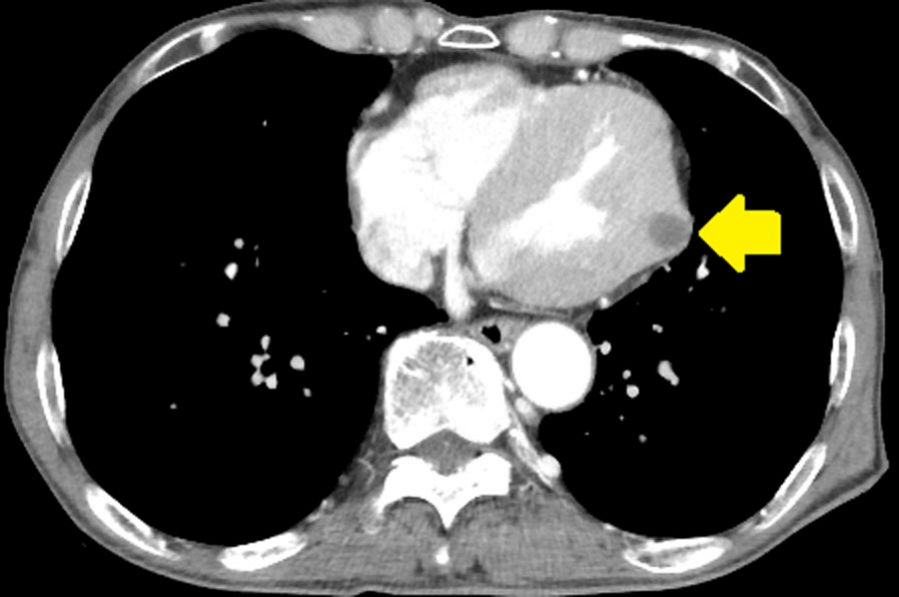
Computed tomography of the chest showing a defect (11 × 11 mm in diameter) in the left ventricle (arrow)
